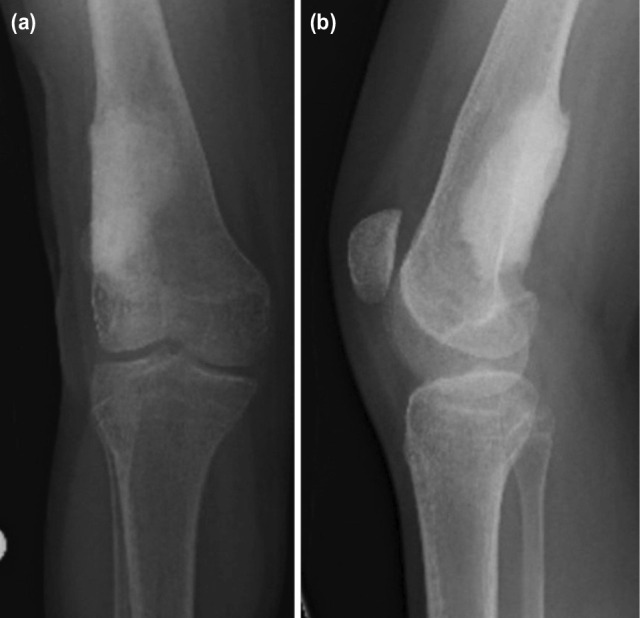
(a) Anteroposterior and (b) lateral radiographs of the right knee at the 3rd year follow-up after plate removal, curettage and cement application. Radiographs show no evidence of recurrence.
